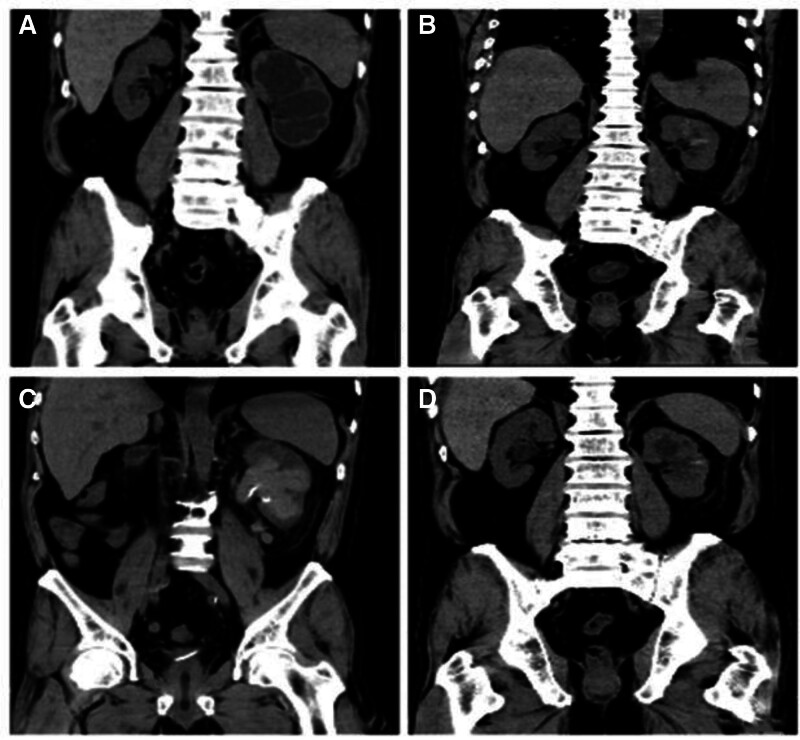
CT changes before and after surgery. (A) Preoperative moderate to severe hydronephrosis of the left kidney. (B) Hemorrhage in the left renal pelvis postoperatively. (C) Significant hematoma in the left renal pelvis after heparinized dialysis. (D) Hematoma in the left renal pelvis has resolved spontaneously before discharge. CT = computed tomography.
